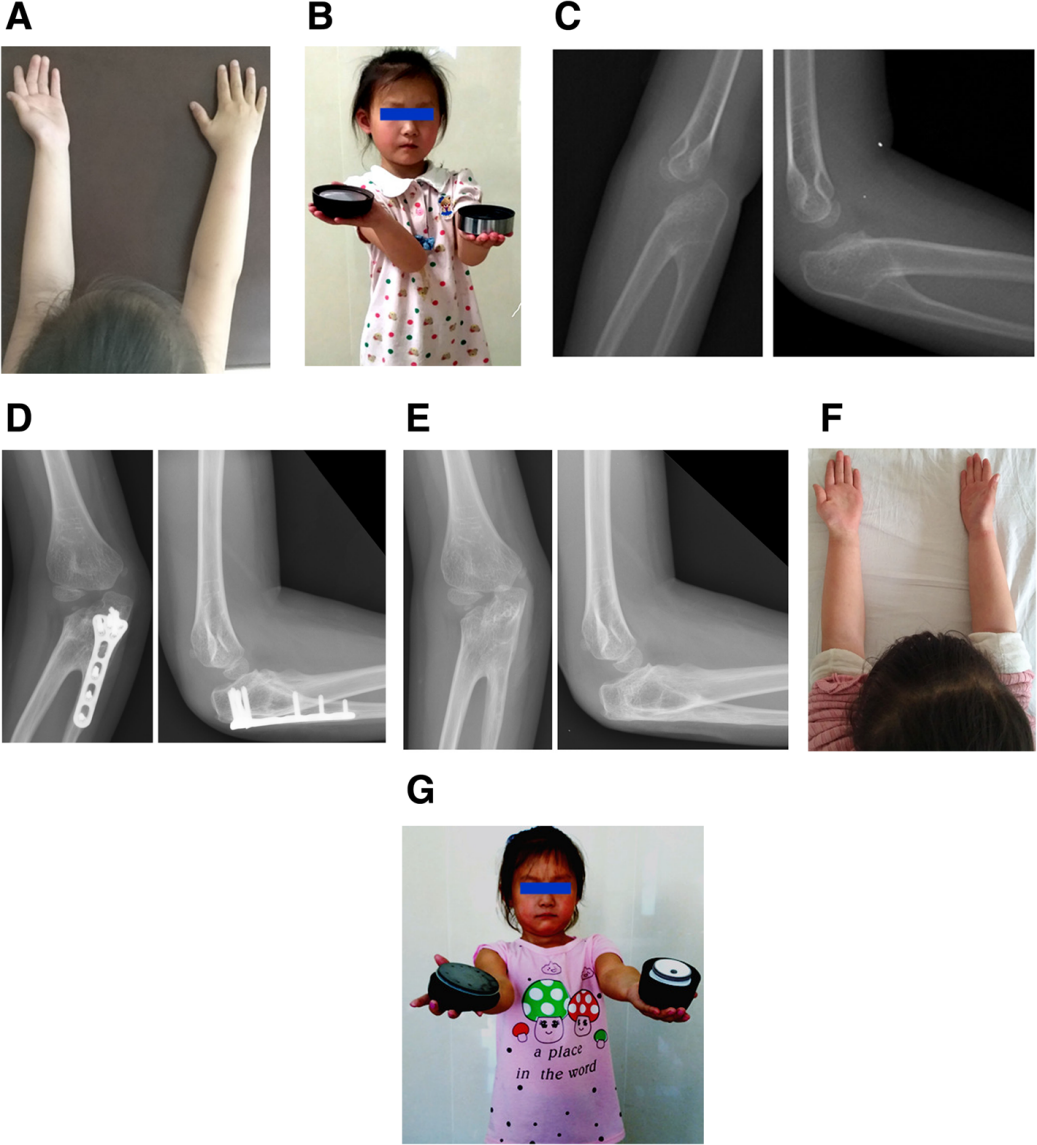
a A 6-year-old girl who had proximal radioulnar synostosis in her right forearm that was fixed in the pronation position. b She experienced difficulty in daily living activities, such as holding a bowl in the palm, washing, dressing, and engaging in personal hygiene care. Her preoperative Failla score was 7 points. c Preoperative anteroposterior and lateral views showing right proximal radioulnar synostosis (type III). d Bone union was achieved at the osteotomy site, and the implants were removed 4 months after surgery. e X-ray showing good remodeling at the osteotomy site at 2 years after surgery. f The rotational function of the right forearm was significantly improved after 2 years. g The patient’s daily activities were greatly improved
